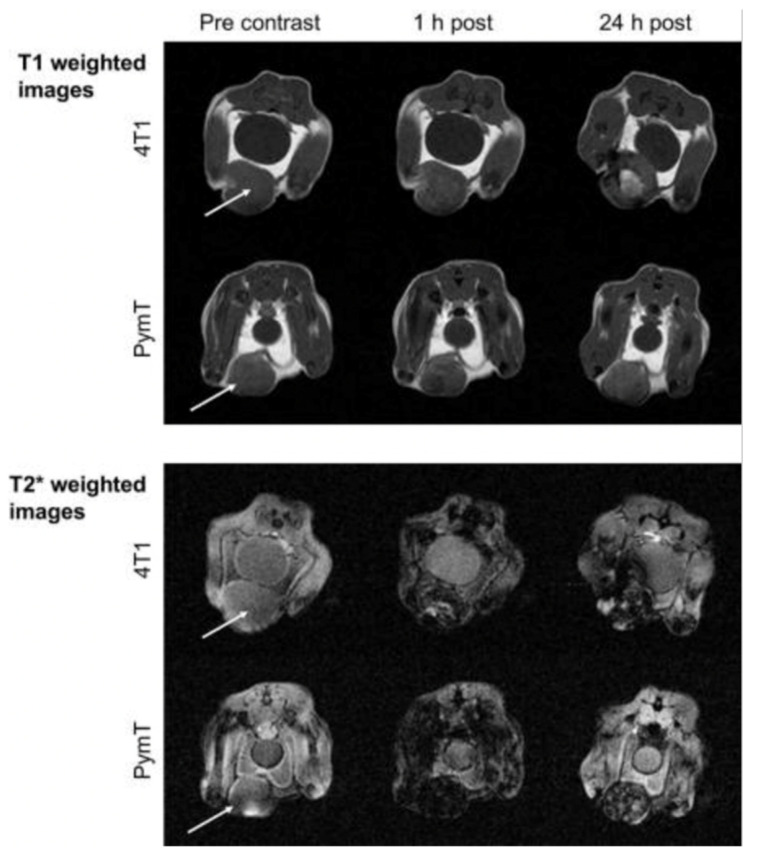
Early necrosis was detected by ferumoxytol in MRI of a fast-growing, aggressive 4T1 mammary tumor and compared with a slow-growing mammary tumor (the MMTV-PymT model). T1- and T2 *-weighted MRI of two representative tumors, pre-injection, 1 h and 24 h post-injection of ferumoxytol (0.5 mmol Fe/kg), showed that both tumors were homogeneous in pre-contrast images, while at 24 h 4T1 tumors exhibited a marked central T1 enhancement. Figures reproduced from ref. [31].
